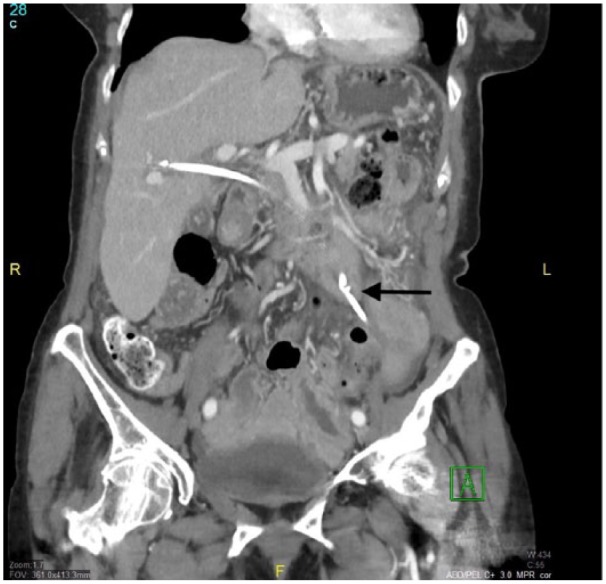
Computed tomography scan of abdomen with contrast showing distal portion of stent traversing luminal wall into peritoneal cavity (arrow).
